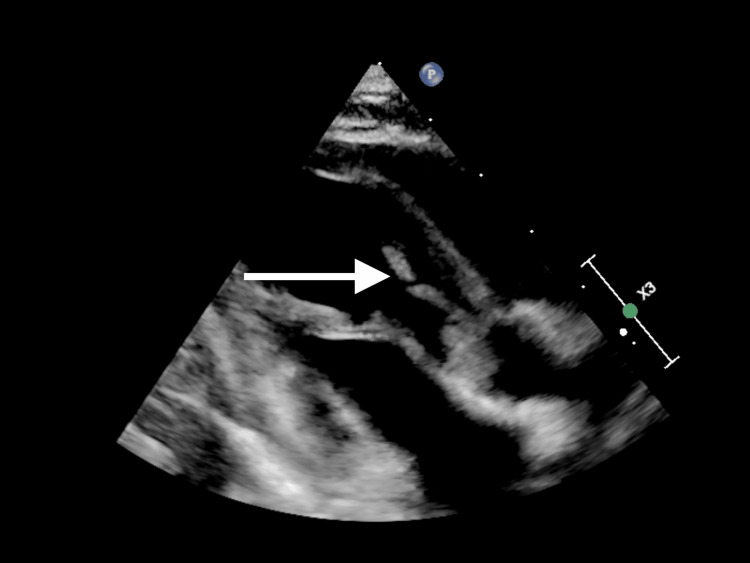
Parasternal long-axis two-dimensional echocardiographic view demonstrates a sizeable vegetation on the aortic valve extending into the left ventricle outflow tract.
